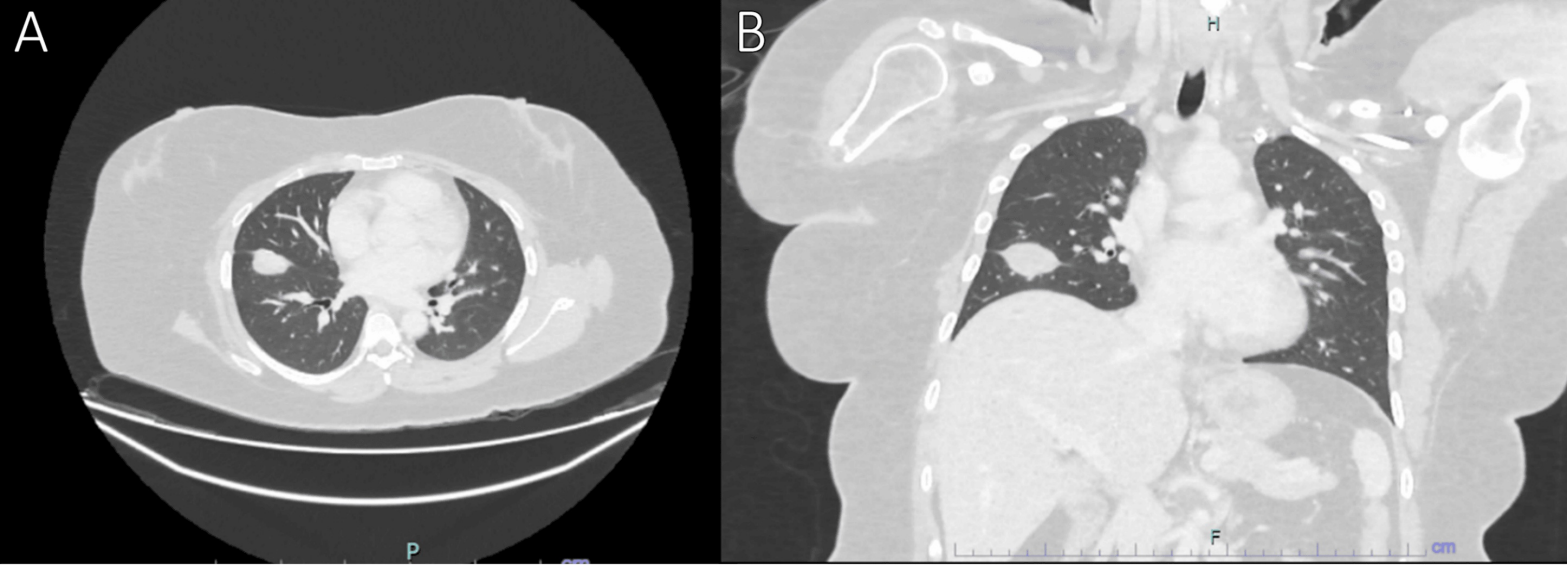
Axial view (A) and the coronal view (B) of the CT scan showed the largest lung mass seen at the right oblique fissure.
